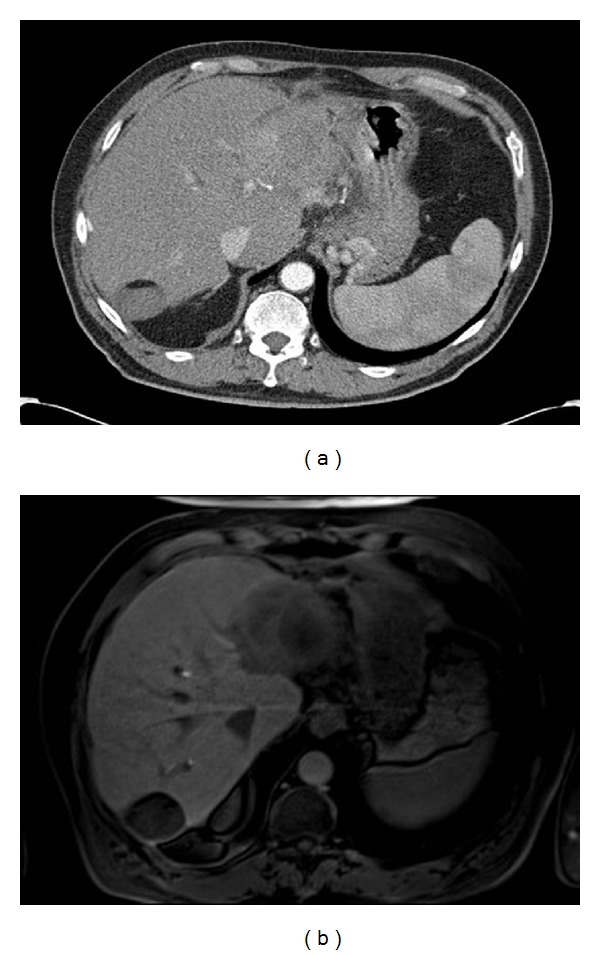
Contrast-enhanced CT compared to Gd-EOB-DTPA-enhanced MRI in the same patient. 69 y. male with colorectal cancer after RFA of segment 6 lesion. New CT and MRI with Eovist ordered for elevated CEA. CT (a) shows a mildly heterogeneous area in segment 2. MRI with Eovist (b) shows a clearly demarcated lesion measuring over 7 cm consistent with metastasis.
